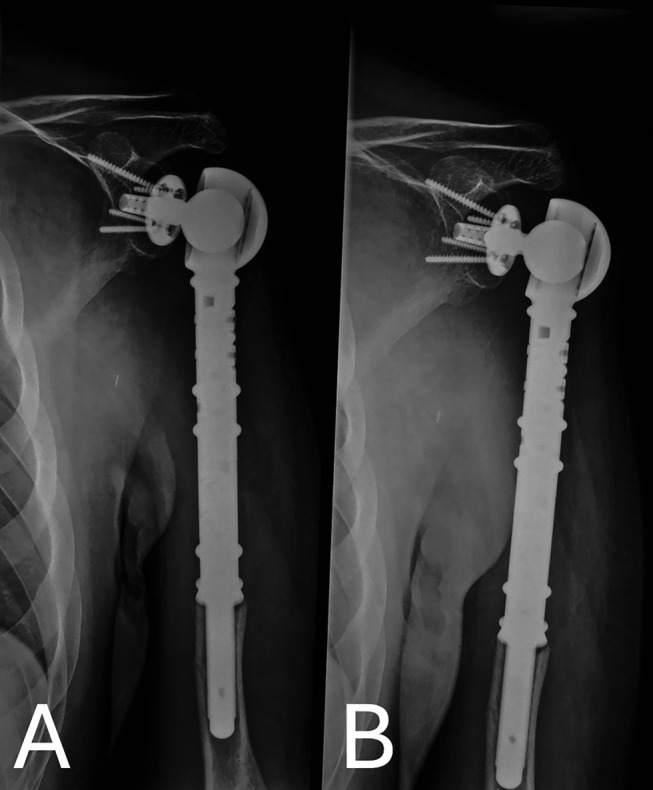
Plain radiograph of the left shoulder at 3.5 years post-operatively showing no signs of loosening or mechanical failure.
